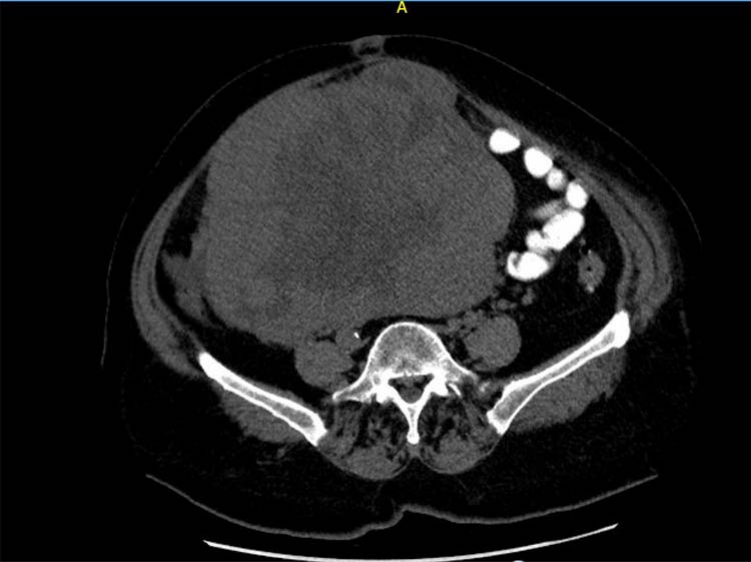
Close picture of axial CT scan of tumor, showing more right-sided localization.
